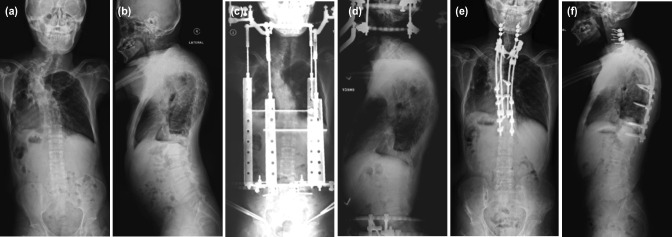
Plain radiographs (posteroanterior and lateral view) of Case 4: (a, b) before operation, (c, d) while on halo-pelvic traction, and (e, f) after single-stage posterior spinal fusion.
